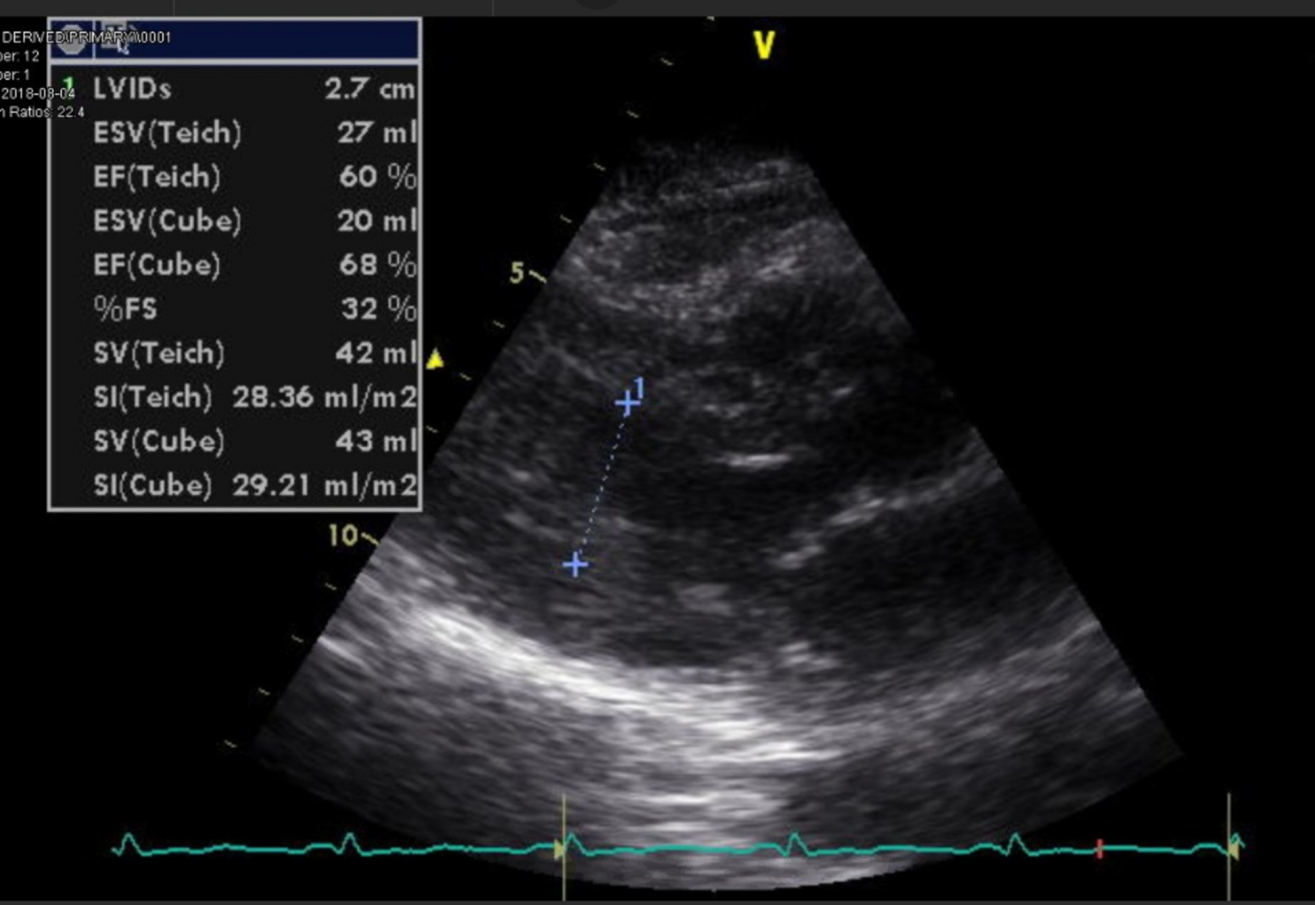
Two-dimensional echocardiogram showing normal biventricular dimensions and left ventricular ejection fraction of 60%.
